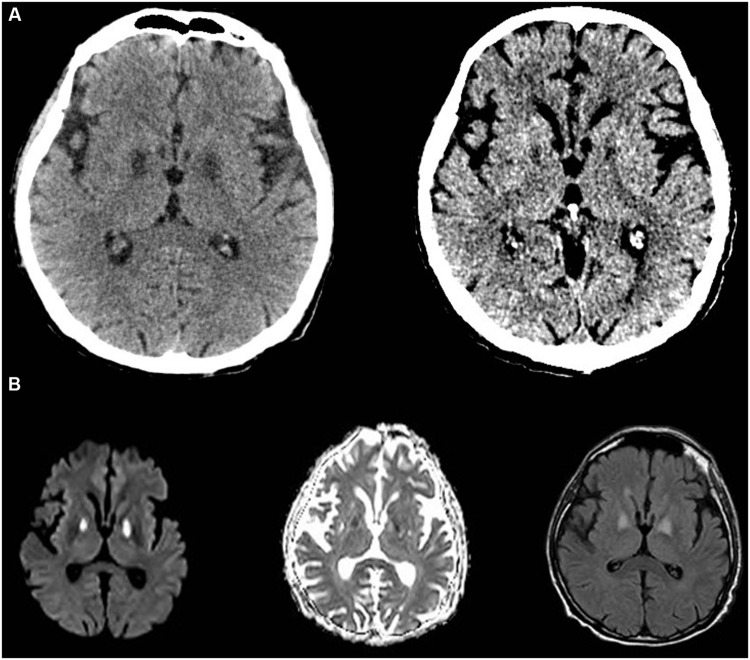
Computed tomography-scan and MRI of the brain. (A) Shows CT of the brain with bilateral globi pallidi hyper-intensities at day 5 (left) and 14 (right) from admission. (B) Shows the MRI images documenting acute ongoing stroke on day 6 on T1, T2, and flair captures, respectively.
